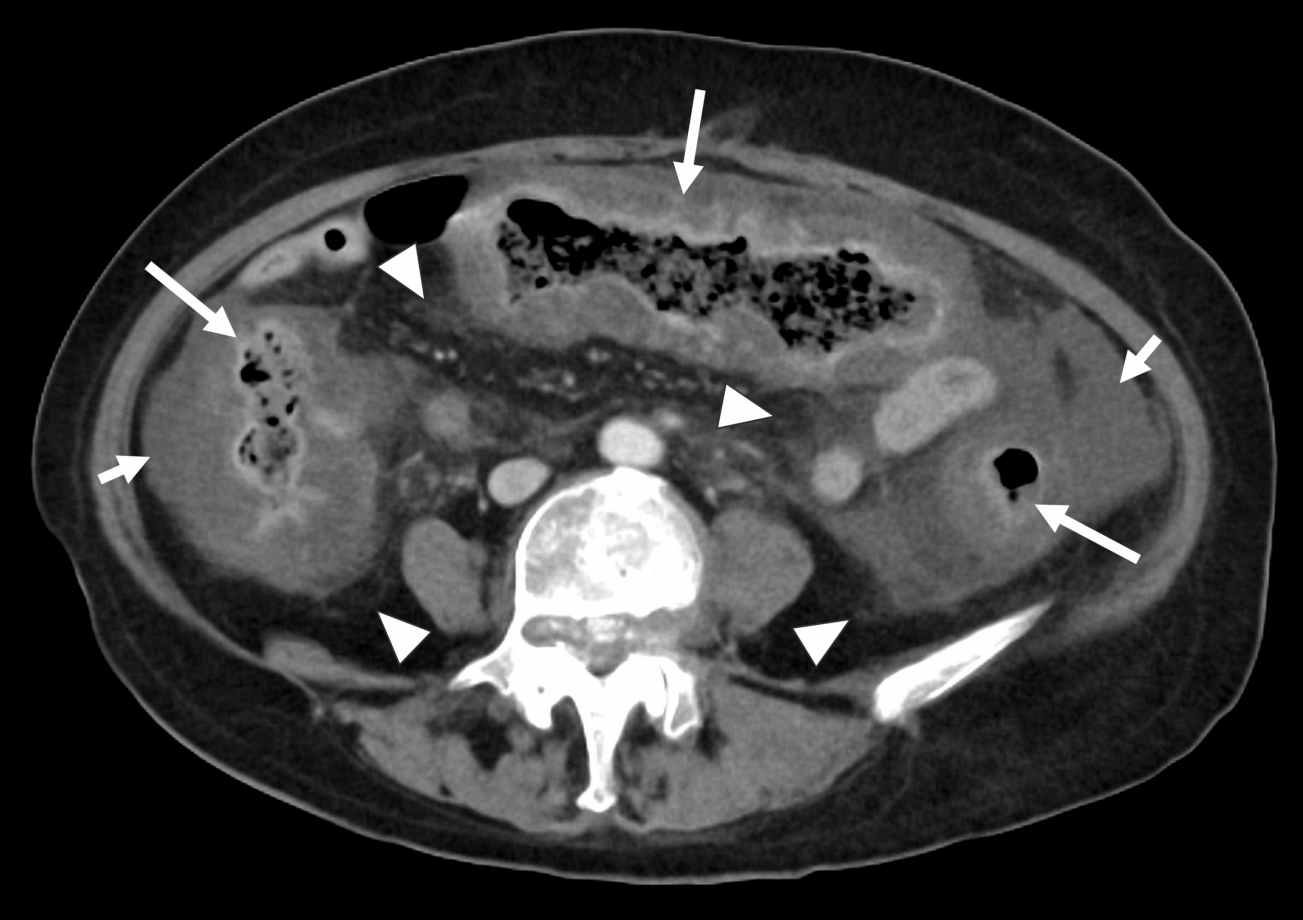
Contrast-enhanced abdominal CT on day 18 revealed marked edematous wall thickening from the rectum to the ascending colon (long arrows), with pericolonic fat stranding (arrowheads) and ascites (short arrows). No perforation or abscess formation was identified. Mucosal enhancement was preserved CT: computed tomography
